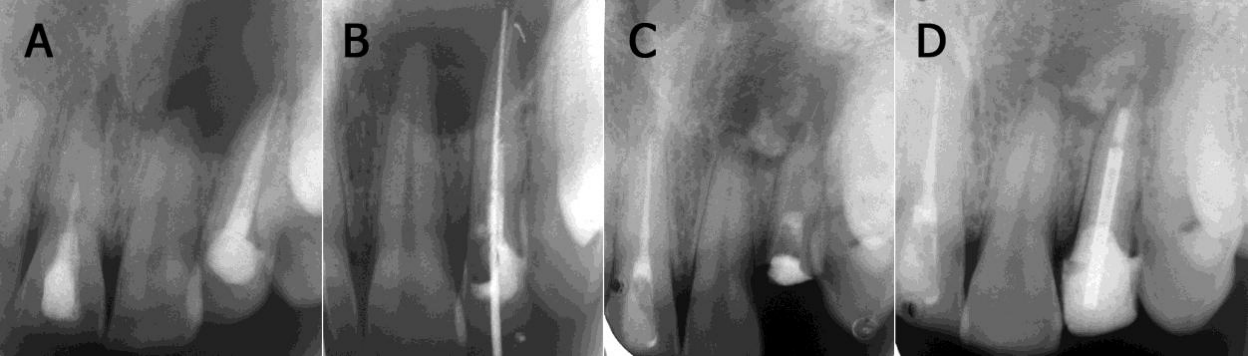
A) Periapical radiograph showing a large periapical radiolucency apparently involving the apices of teeth #9 and 10. Tooth #10 shows a radiopaque material extending beyond the radiographic apex by approximately 2.5mm. B) Periapical radiograph showing gutta-percha beyond the root apex which could not be retrieved and a no. 40 hand K-file that passed beyond the apical foramen. C) Periapical radiograph after 5 months showing a favorable amount of periapical healing. The weakened coronal tooth structure was removed. D) Periapical radiograph after 1 year 3 months showing considerable amount of periapical healing in spite of the overextended gutta-percha
